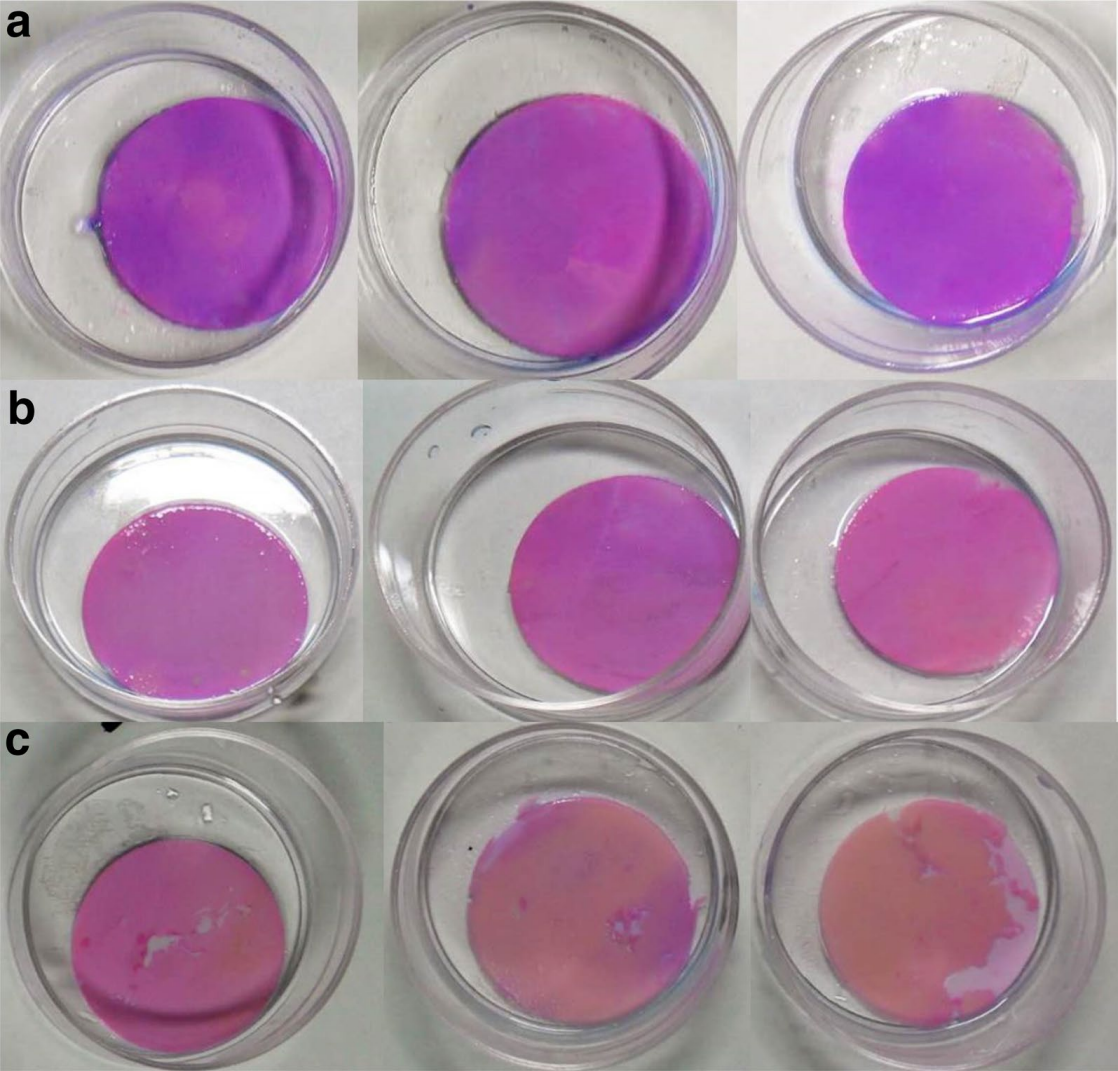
The comparison of the destruction degree of samples under different treatment conditions. a is the control group. From left to right, only 1 mL saline is incubated for 1.2.3 min, respectively. B is the sinusoidal ultrasound group. From left to right, 1 mL saline is incubated for 3 min and the intensity of sinusoidal ultrasound is 0.5, 1 and 1.5 W/cm^2^, respectively. c is the pulse ultrasound group. From left to right, 1 ml saline is incubated for 3 min and the intensity of pulse ultrasound is 0.5, 1 and 1.5 W/cm^2^, respectively.
